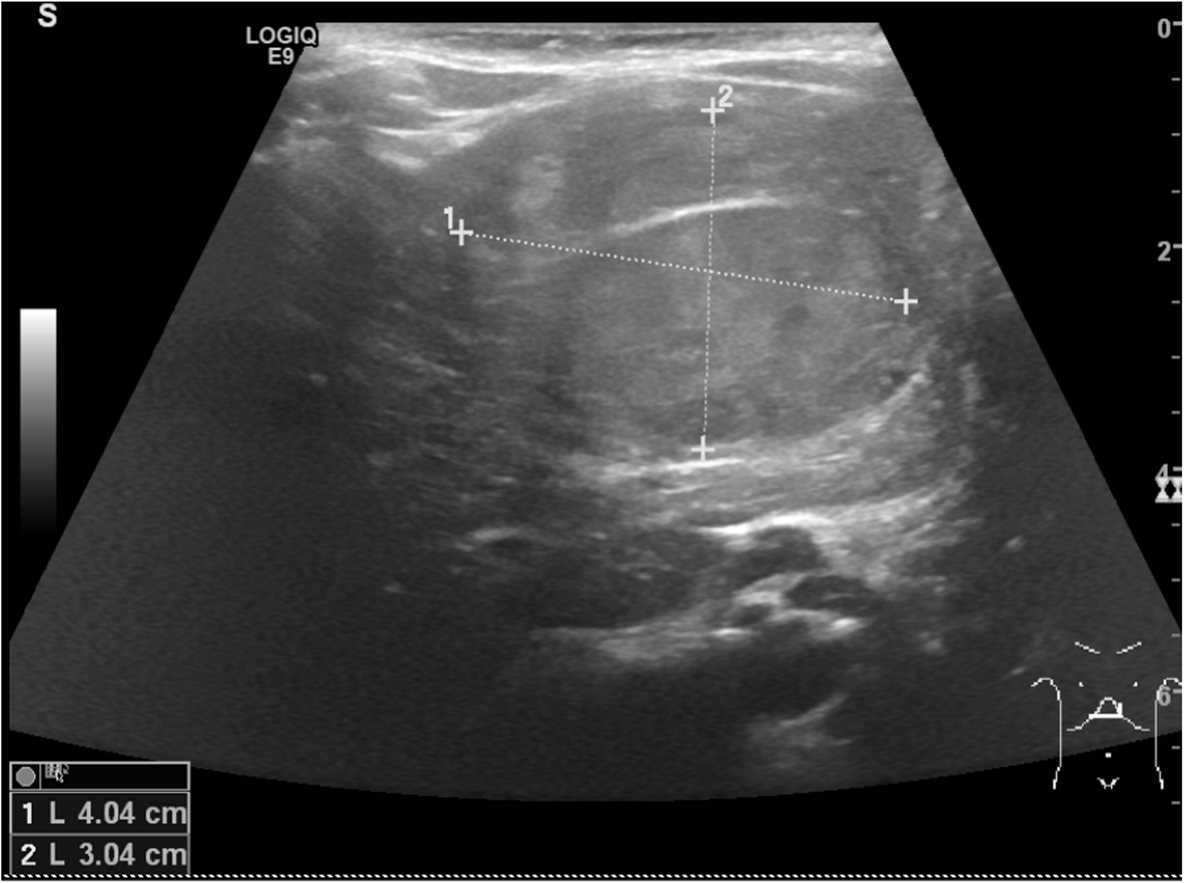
Abdomen ultrasound scan of the patient’s liver showing the hepatoblastoma. The figure shows a scan of the abdominal ultrasonography of the patient: it revealed a solid mass of 4 × 3 cm in the left lobe of the liver, with well-defined edges, ovular and bilobate shape
